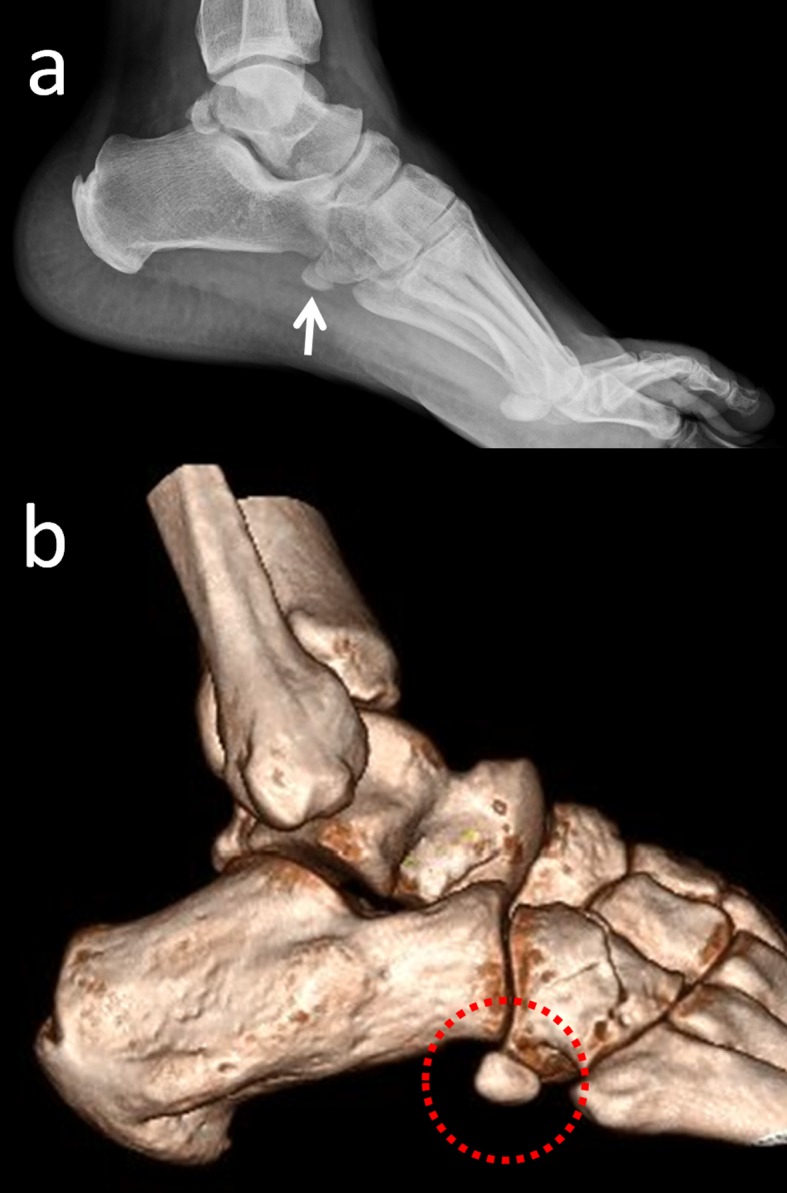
Appearance of os peroneum in a patient with cuboid fracture (a) Lateral foot radiograph; (b) 3D computed tomography (CT) appearance.
